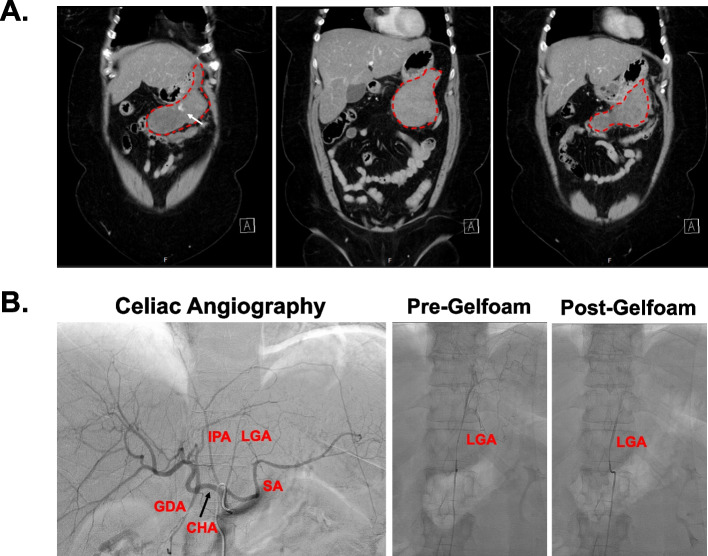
A 43 months after primary resection, patient presented with epigastric abdominal pain and tachycardia. Multiple coronal slices of CT demonstrate ~ 6.8 x 6.1 x 5.4 cm mass along greater curvature of the stomach (red outline) with associated active extravasation (white arrow). B Angiogram and gelfoam embolization of left gastric artery (LGA). Celiac angiography identifies diminutive splenic artery (SA), left gastric artery (LGA), inferior phrenic artery (IPA), gastroduodenal artery (GDA), and common hepatic artery (CHA)
